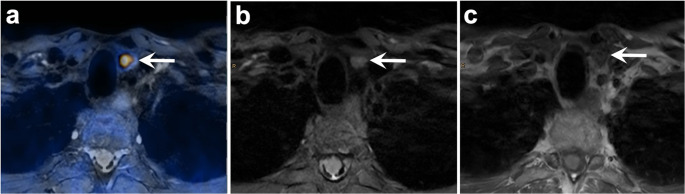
48 year-old man with pHPT (PTH level 123.0 pg/mL, albumin-corrected serum calcium level 2.67 mmol/L). An orthotopic left-sided lower pole parathyroid adenoma (arrow; SUV_max_ 9.4, 8.0 x 5.5 x 11.0 mm) is seen on axial fused [^18^F]fluorocholine PET/MR image (a), axial T2-weighted flex water MR image (b) and axial T1-weighted FSE MR image (c)
